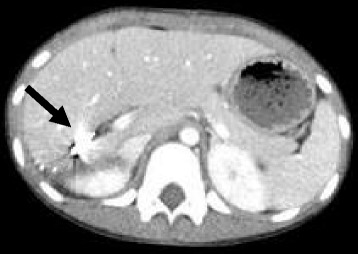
An axial view of an enhanced abdominal computed tomography (CT) scan on the 14th day after extended right lateral sectionectomy shows no evidence of an intra-abdominal abscess and biloma. Embolized coils (*arrow*) are also observed
